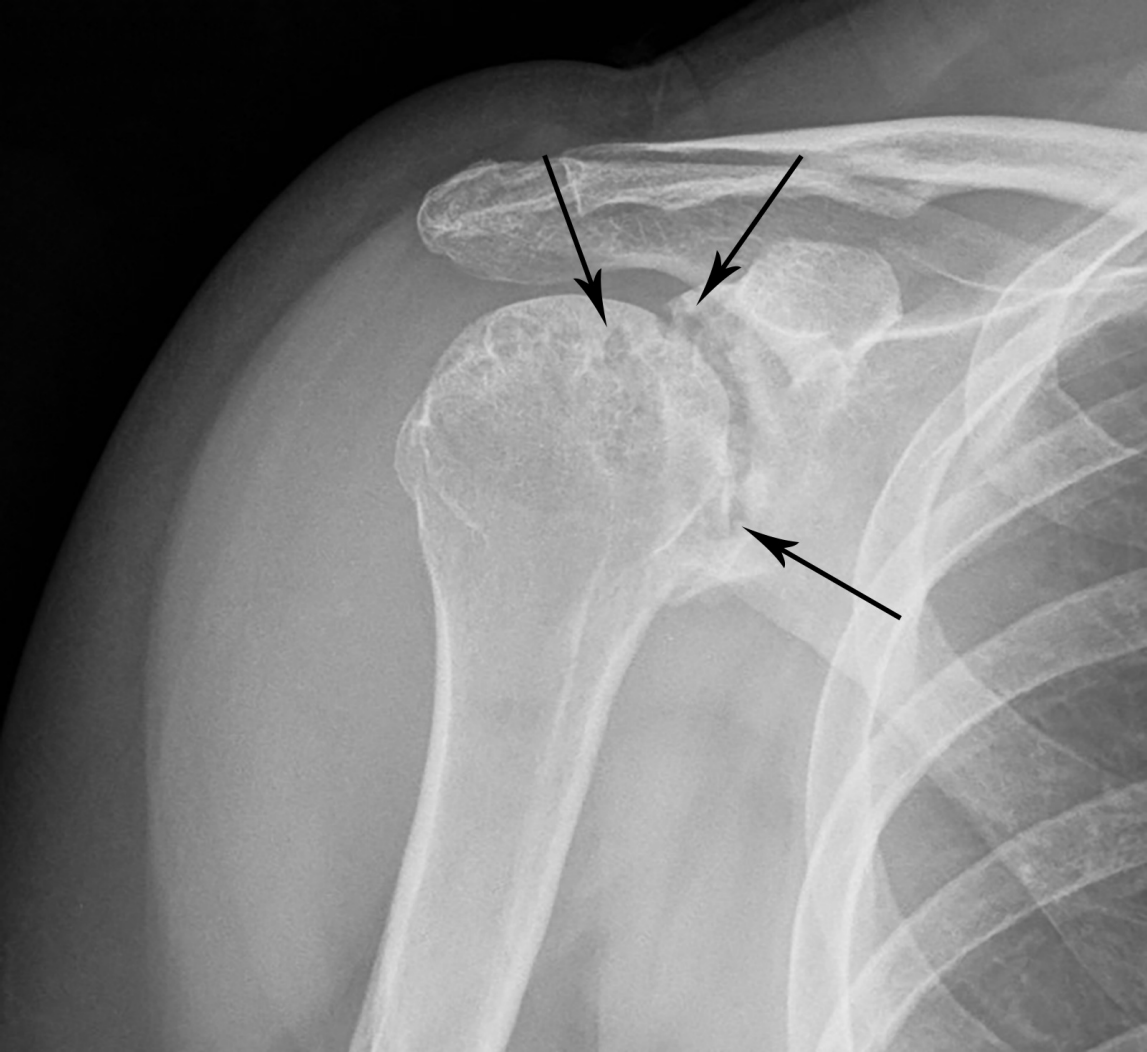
Radiograph of the Right Shoulder in this 28-Year-Old Female with Rheumatoid Arthritis. There are numerous periarticular joint erosions (arrows) and joint space narrowing which is consistent with diagnosis of with rheumatoid arthritis.
